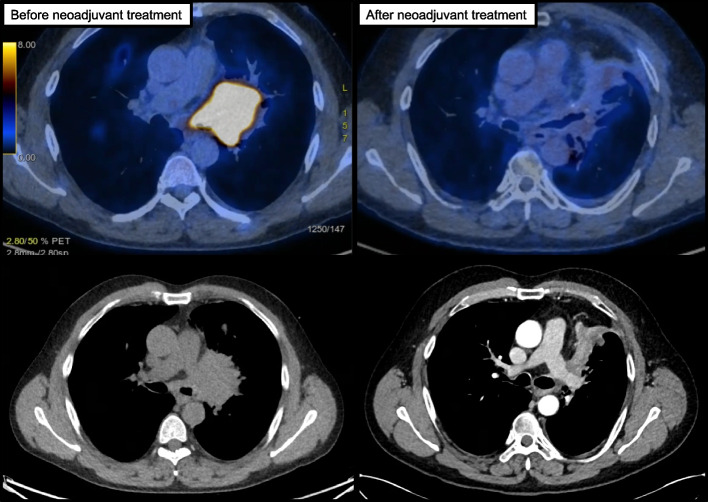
Positron-emission computed tomography (PET-CT) and computed tomography (CT) before initiation of neoadjuvant treatment (left) and after administration of neoadjuvant chemoradiotherapy and immunotherapy (right). The contrast-enhanced CT (left, below) depicts the left main pulmonary artery encircled by the residual tumor mass
